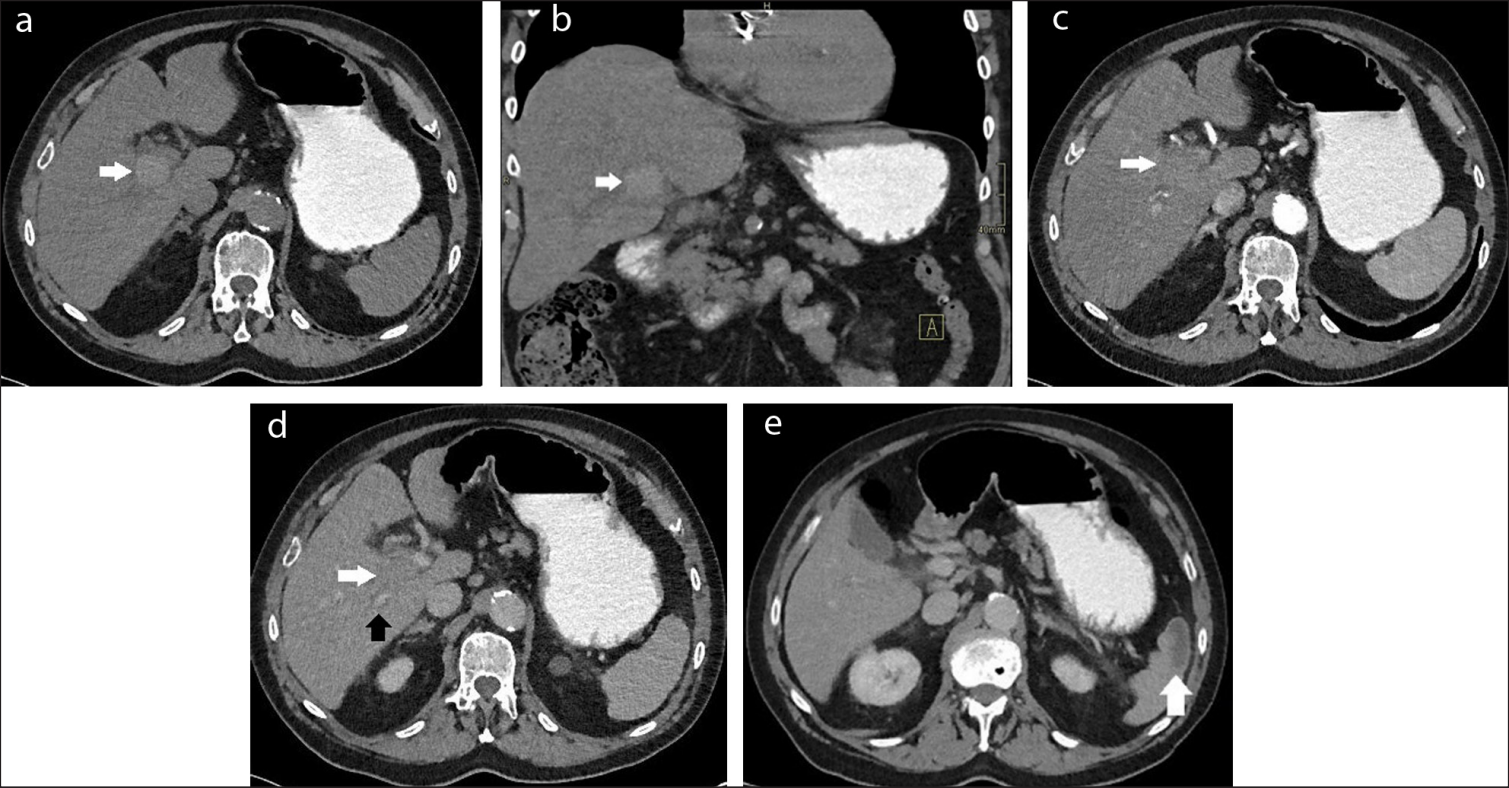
Axial (a) and coronal (b) unenhanced CT of the upper abdomen shows a homogenous spontaneous hyperdense ovoid lesion of 3.2 cm (white arrow). The arterial (c) and venous (d) phase of the contrast enhanced CT shows no enhancement, resulting in hypodense appearance relative to the liver. Compression of the right portal vein (d, black arrow) is depicted. In addition, a segmental splenic infarction is seen (e, white arrow).
